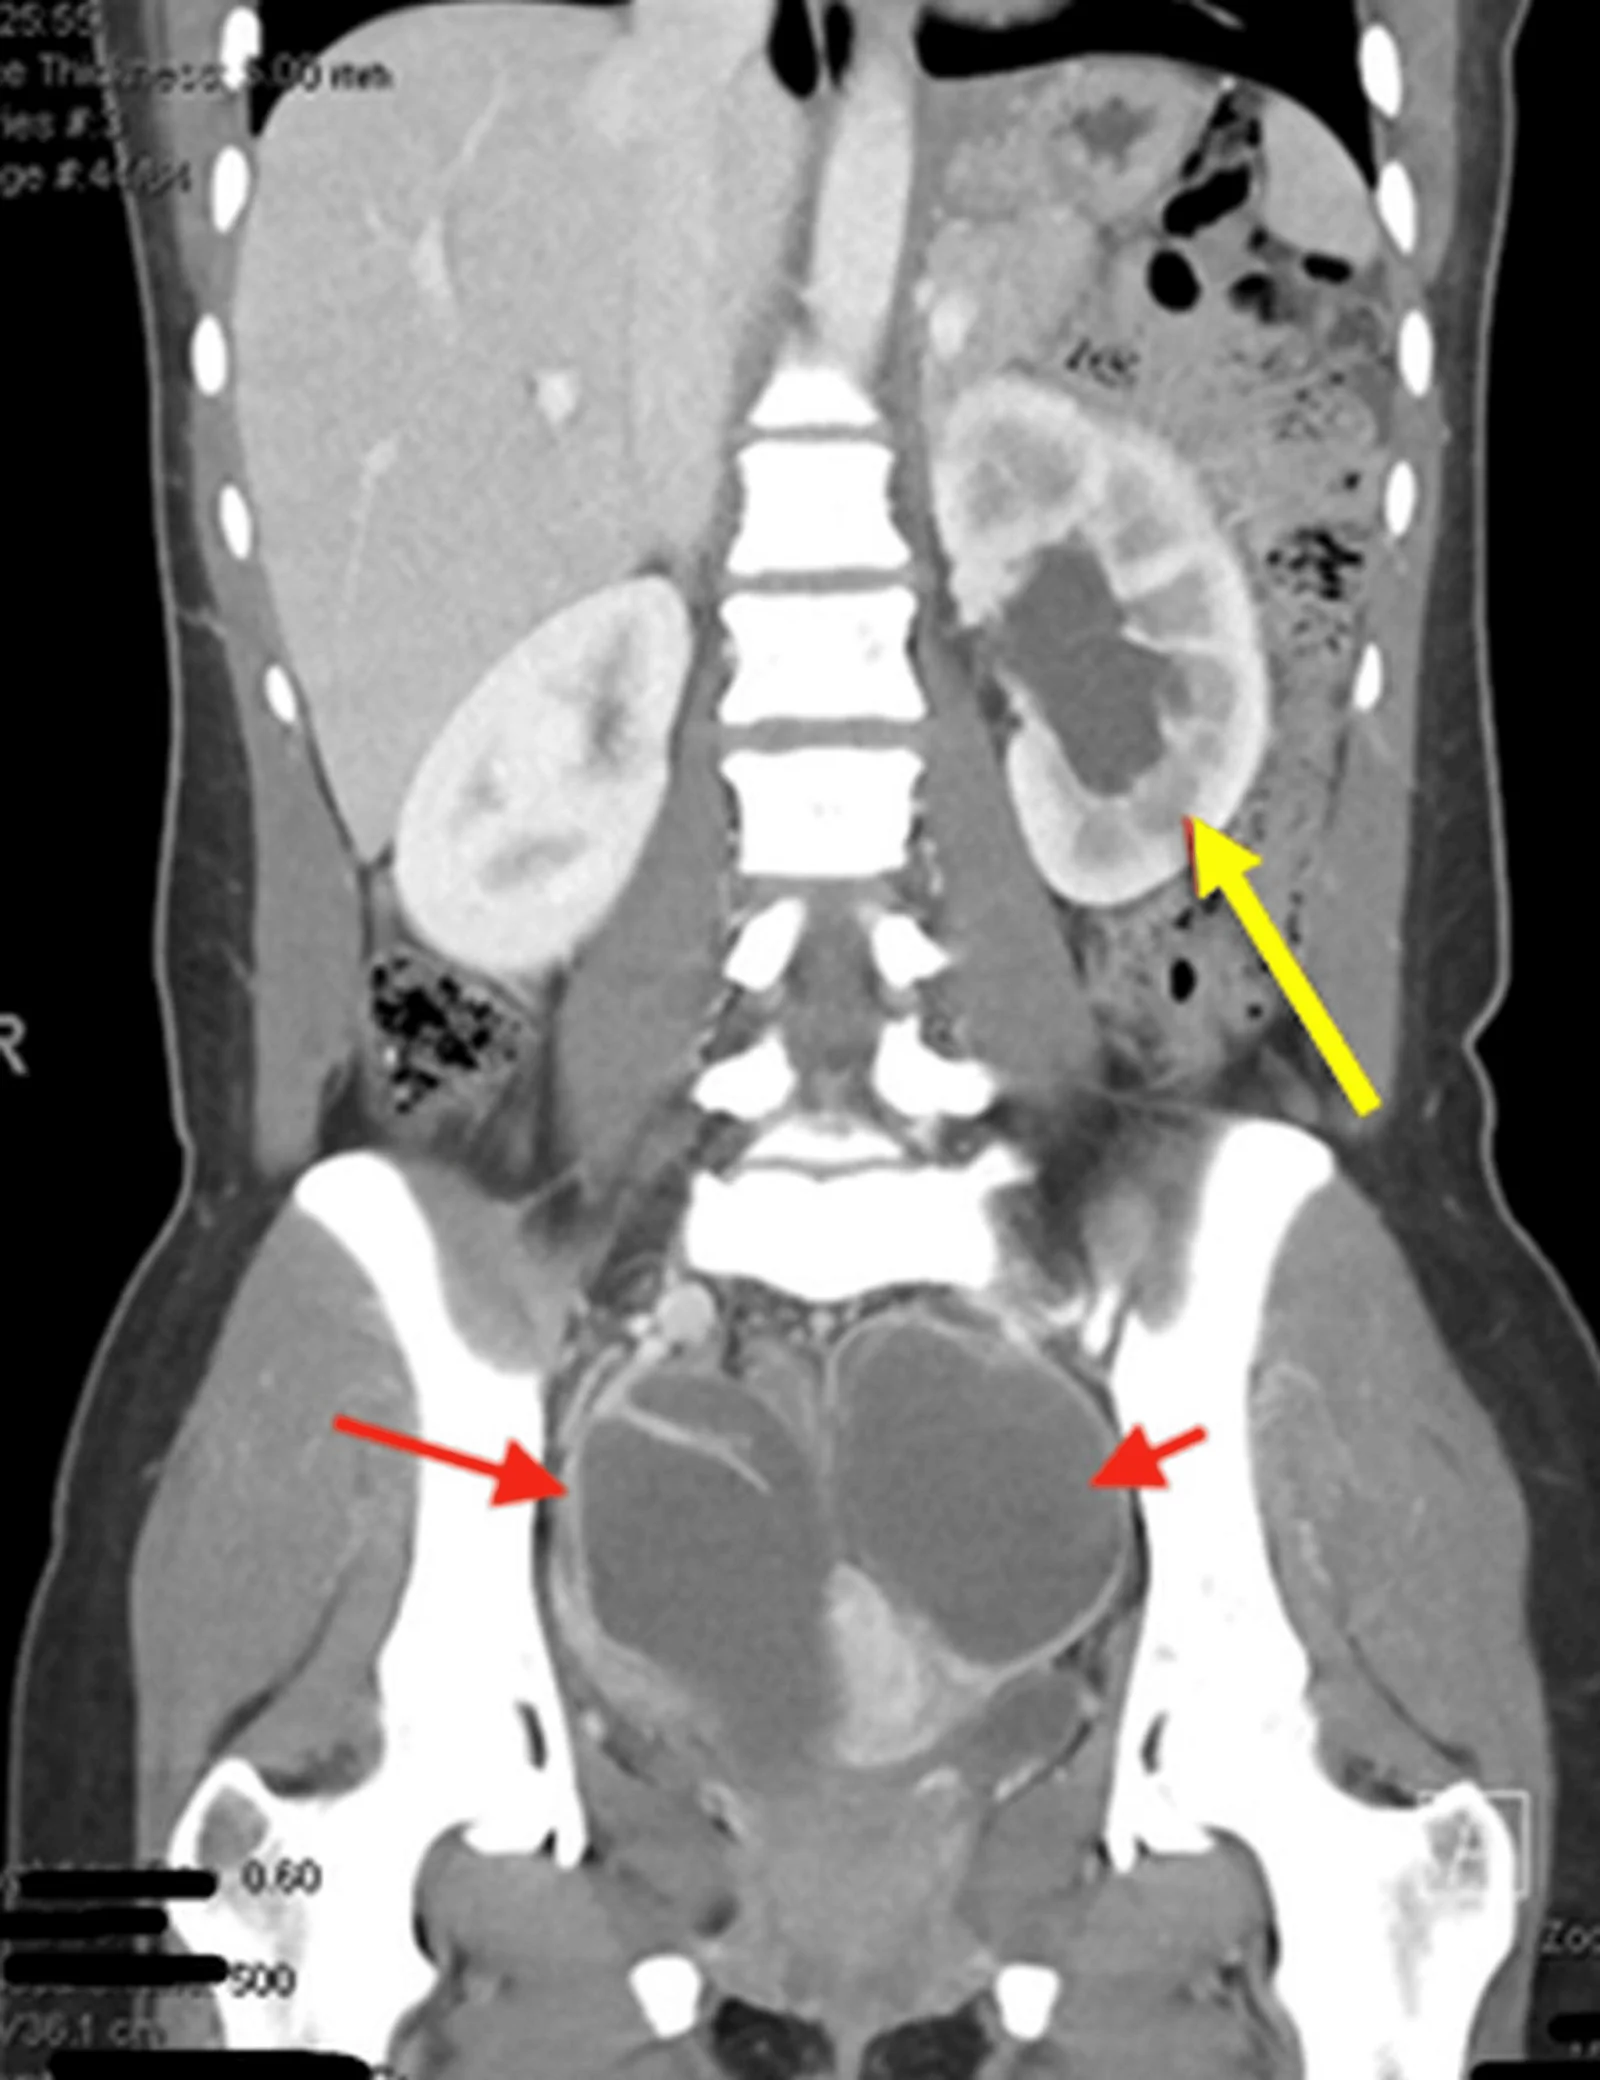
Contrast-enhanced CT demonstrating left hydronephrosis and pelvic mass effect Renal changes presumed to be secondary to severe bilateral hematosalpinx. The findings suggest that the obstruction is extrinsic rather than due to an internal urinary calculus. The lower red arrows indicate two large, distinct fluid-filled pelvic masses that appear to compress the ureters externally, resulting in impaired urinary drainage. These masses are favored to represent hematosalpinx rather than ovarian pathology, including endometriomas. CT: computed tomography
